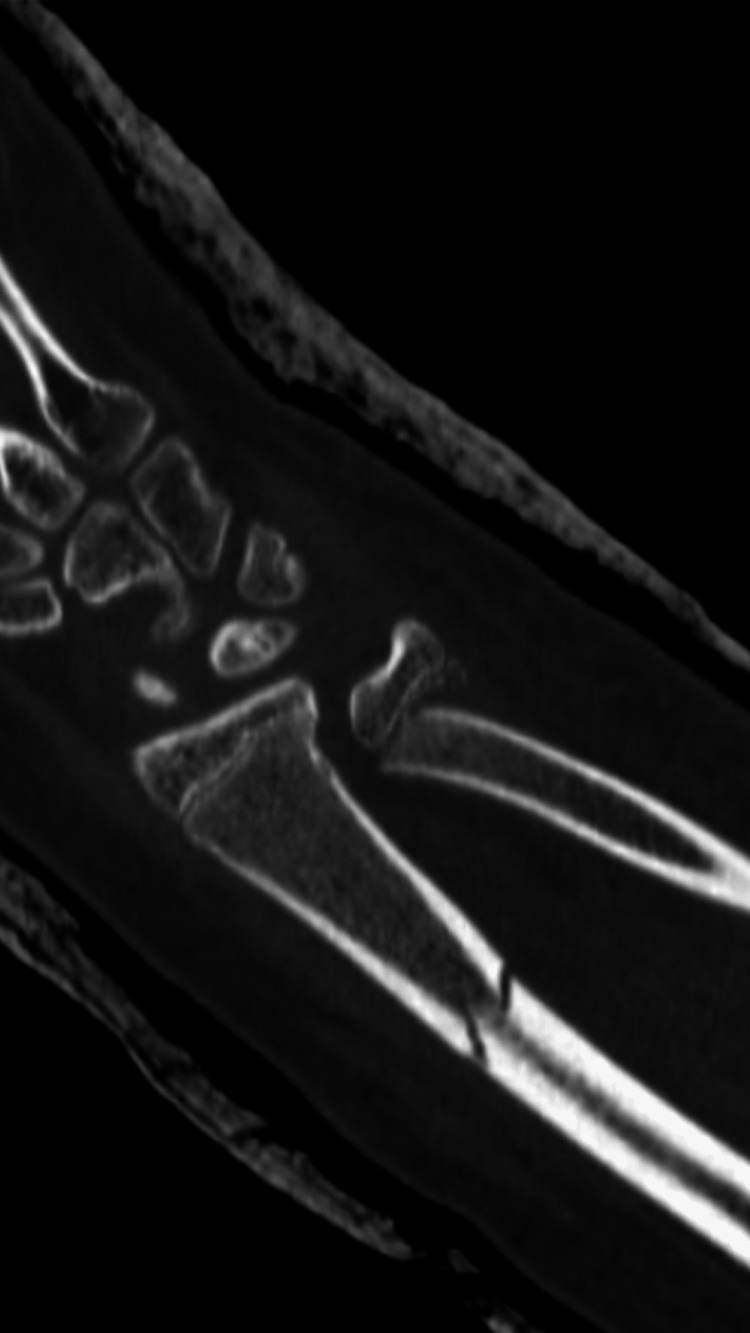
CT imaging of the left wrist in the coronal plane. CT: computed tomography.
